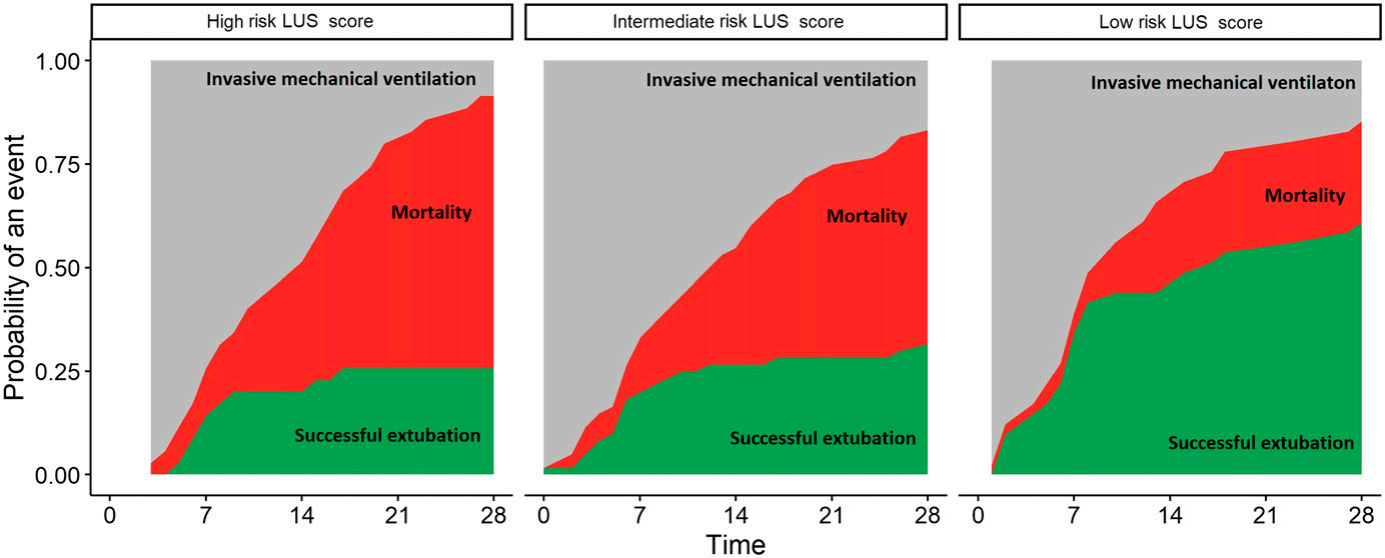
Three categories of global lung ultrasound (LUS) score and cumulative incidence of outcomes. x-axis: days since intubations; y-axis: probability of an event (extubation or death) in the population. The three facets show the risk for patients with a high risk global LUS score (left), intermediate risk (middle) and low risk global LUS score (right). Red area show the patients who died. Green area shows the patients who were successfully extubated. This figure appears in color at www.ajtmh.org.
